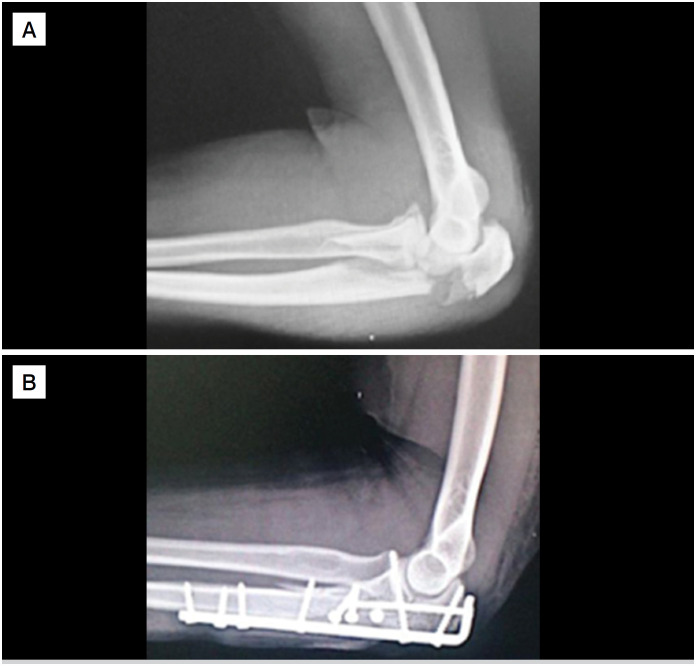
A and B: Pre and Postoperative radiograph of the profile views of the participant's left elbow showing transolecranon fracture-dislocation (A) and osteosynthesis with a 3.5 mm non locking compression plate, and lag screws (B).
